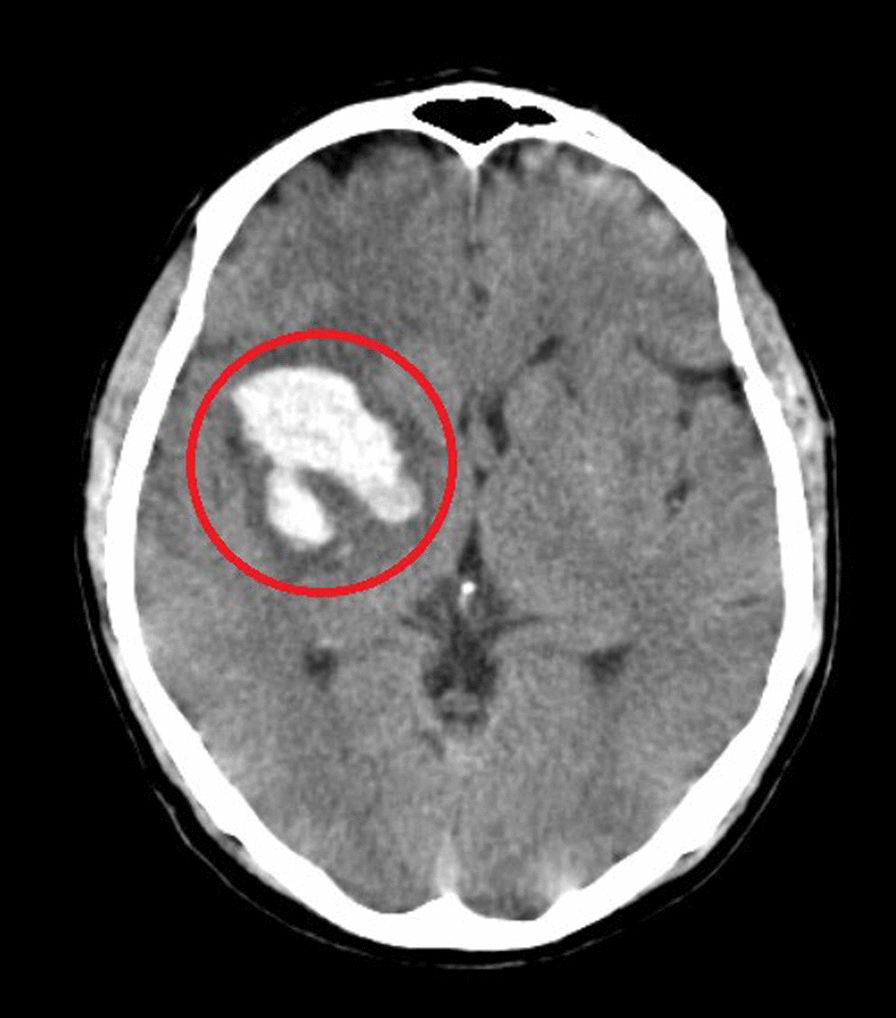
CT brain showing a moderate size of bleed in right gangliocapsular region with involvement of the internal capsule, obligating to the anterior pole of the right lateral ventricle
